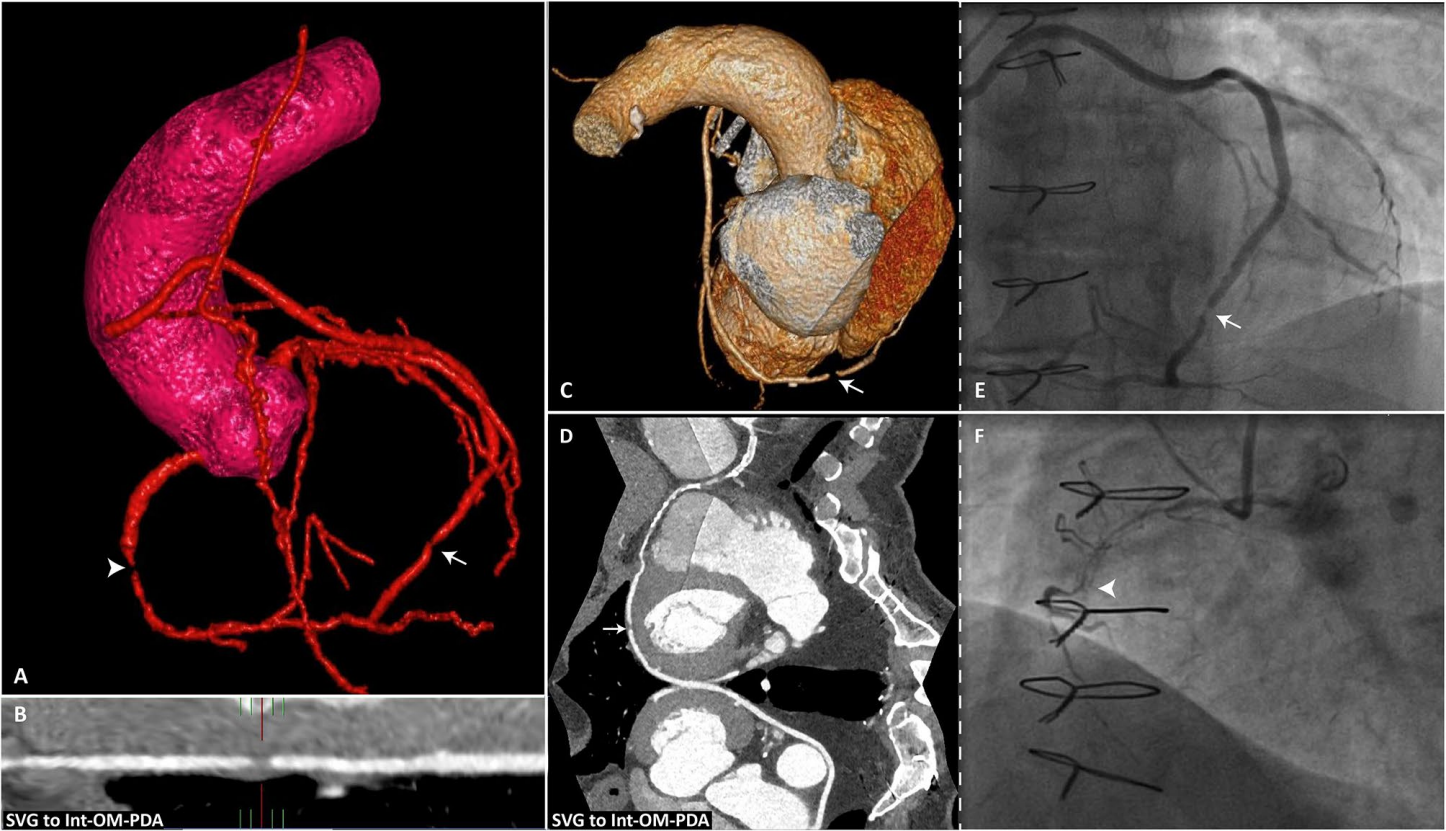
CCTA to evaluate bypass graft failure. CCTA and subsequent invasive coronary angiography images in a post-CABG patient with recurrent angina symptoms. A–D CCTA reconstructed images showing a significant lesion in a saphenous vein jump graft with distal anastomoses on the intermediate branch, an OM branch and the PDA. The lesion is located between the anastomosis on the OM branch and the PDA (white arrows). In addition, a CTO was found in the RCA, depicted in the 3-dimensional reconstruction image in (A), indicated with the white arrowhead. E Subsequent angiography images confirmed the lesion in the venous jump graft (white arrow) (F) and in stent occlusion of the RCA (white arrowhead). CCTA coronary computed tomography angiography, CTO chronic total coronary occlusion; other abbreviations as in Fig. 1
